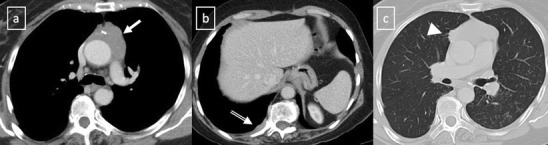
CT is the imaging modality of choice for evaluating staging thymoma. Stage IVa thymoma (WHO type B3) in a 69-year-old woman. a, b Contrast-enhanced CT scan shows a well-circumscribed, flattened soft tissue lesion in the anterior mediastinum with calcification (*arrow*). Note the lobulated contour of the mass and the loss of the fat plane between the mass and the aorta. Pleural seeding is identified as an enhancing pleura-based nodule (*open arrow*). c Irregular border between the mediastinal mass and the lung parenchyma (*arrowhead*) is observed as a sign of locally advanced disease. Note the cellular bronchiolitis in the left lower lobe
